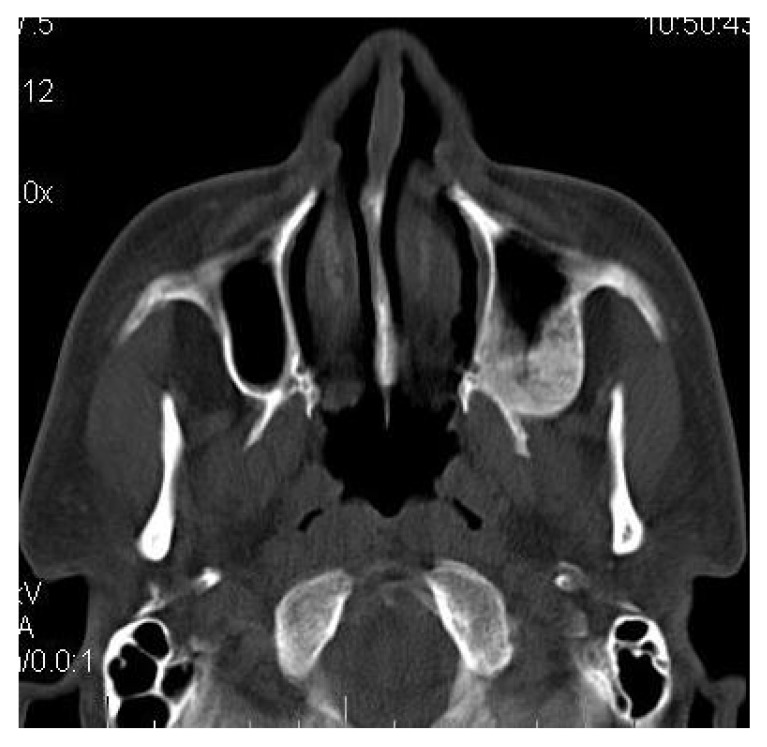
Axial CT section revealing the involvement of the left maxillary sinus and base of the left pterygoid plate.
